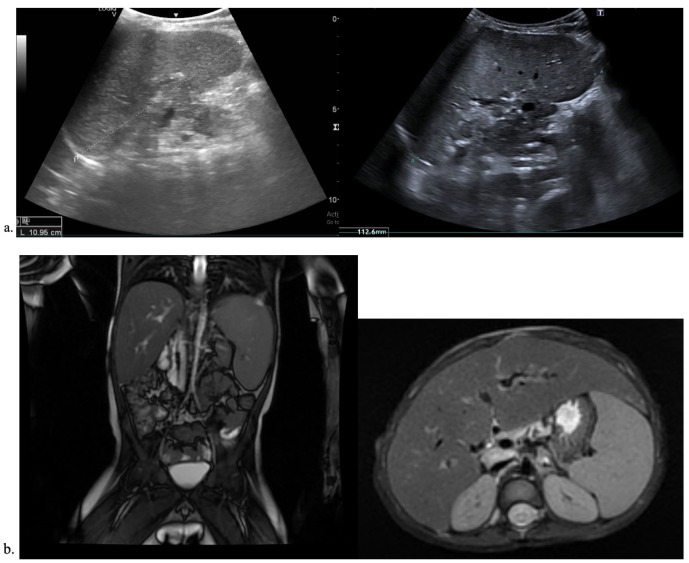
Imaging findings in case 3. (a) Abdominal US—hepatosplenomegaly. (b) Abdominal MRI—hepatosplenomegaly.
